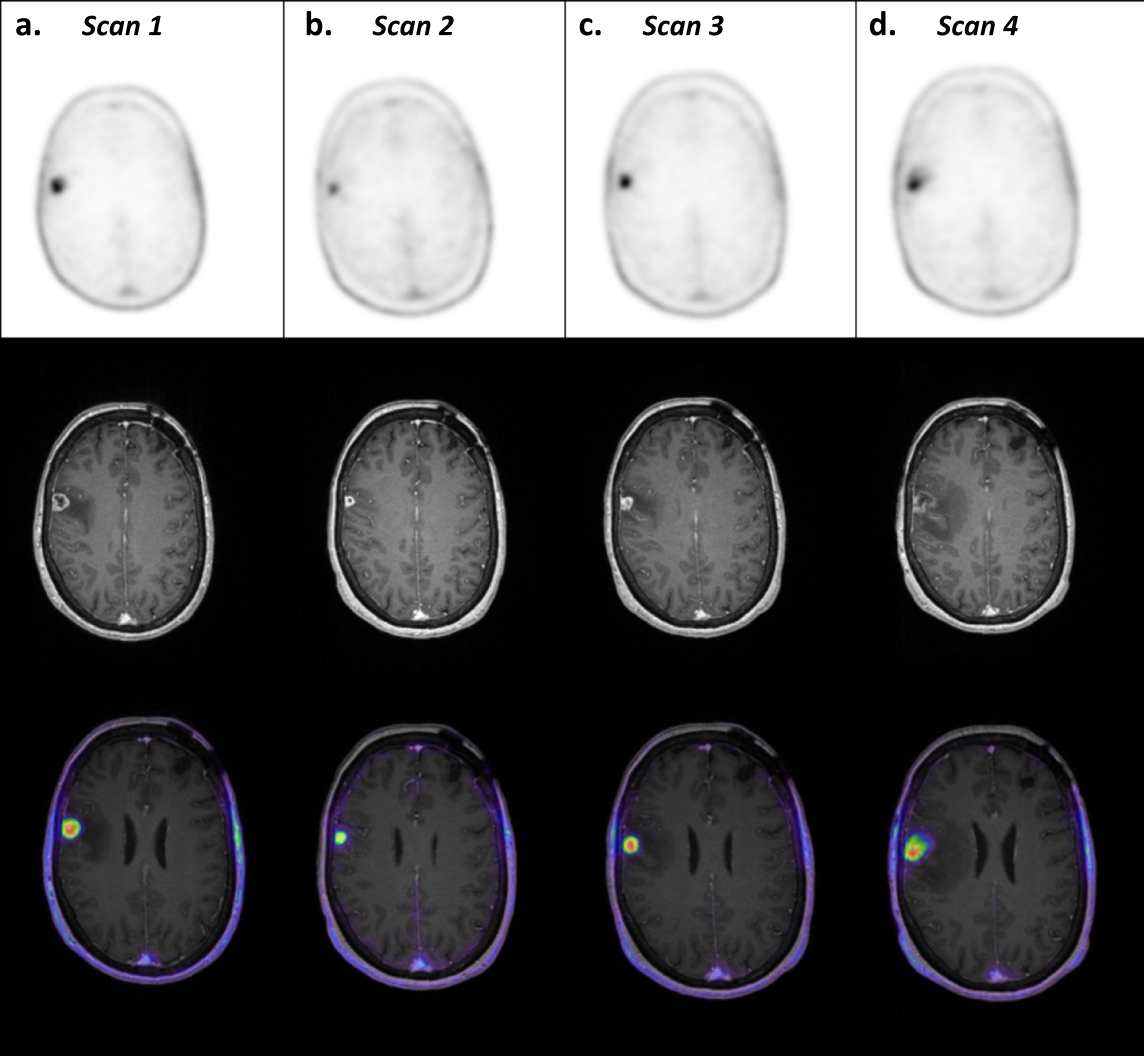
Static PET, T1 MRI_CE_ and fused PET/MR images of the cerebral tumour from scans 1–4. Axial view of the patient’s metastasis in the lateral precentral gyrus of the right hemisphere acquired using hybrid PET/MR imaging at four time points: scan 1—before stereotactic radiation, scan 2—1 month after the end of stereotactic radiation, scan 3—on suspicion of recurrence 1 month after scan 2, and scan 4—1 month after scan 3. Static PET (20–35 min), T1 MRI_CE_ and fused PET/MR images are shown from the top to bottom rows, respectively. Columns a–d show scans 1–4. The SUV scale for static PET images was 0–3.3, and the SUV scale of fused PET/MR images was 0.45–3.3
